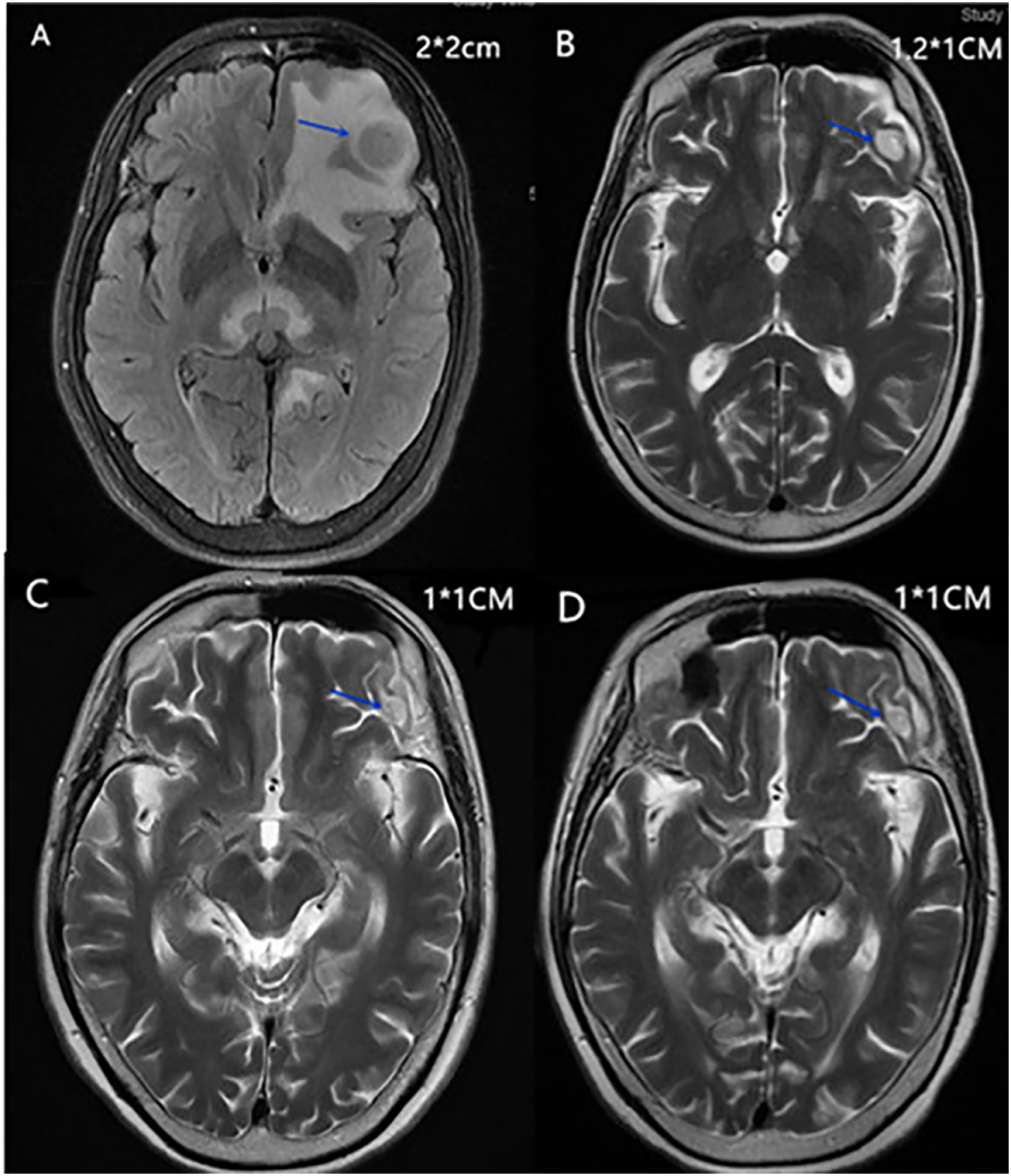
(A) Head MRI of the maximal mass in the left frontal lobe of the brain in April 2020. (B) Head MRI of the maximal mass in the left frontal lobe of the brain in May 2020. (C) Head MRI of the maximal mass in the left frontal lobe of the brain in August 2020. (D) Head MRI of the maximal mass in the left frontal lobe of the brain in April 2022.
